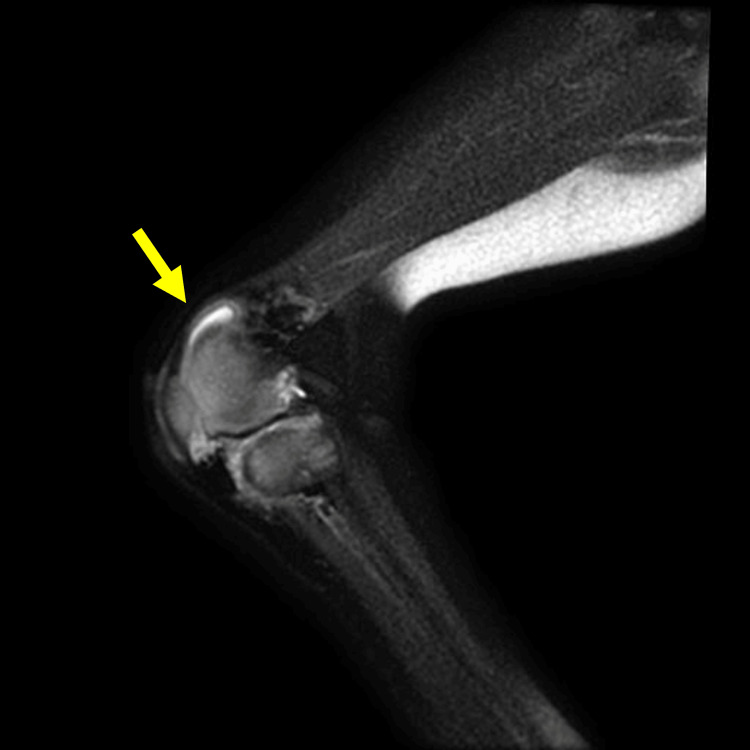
Magnetic resonance imaging of the knee joint The magnetic resonance imaging (T2-weighted image) of the right-side knee joint was shown (yellow arrow). The enhanced T2 signal of the synovial membrane indicated inflammation, suggesting a possible diagnosis of juvenile idiopathic arthritis. Similar findings were also identified in the left knee joint.
